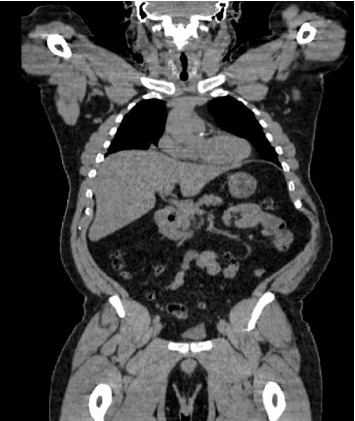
CT scan findings. Coronal contrast-enhanced CT scan of the chest showing multiple bilateral pulmonary nodules of varying sizes. Findings initially raised suspicion for metastatic disease in a patient with a history of renal cell carcinoma.
